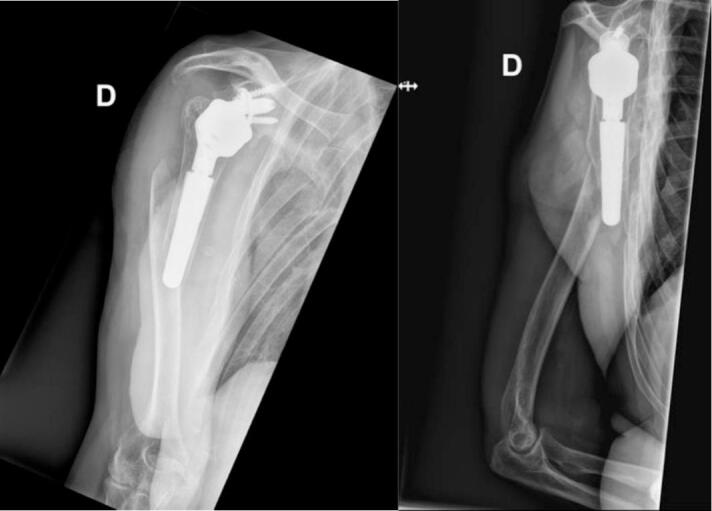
Initial X-rays showing the periprosthetic humeral shaft fracture.
